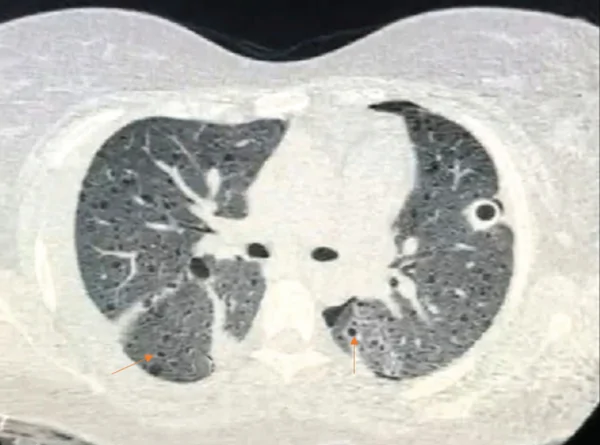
CT scan after placement of chest tube (arrows showing the cysts).
